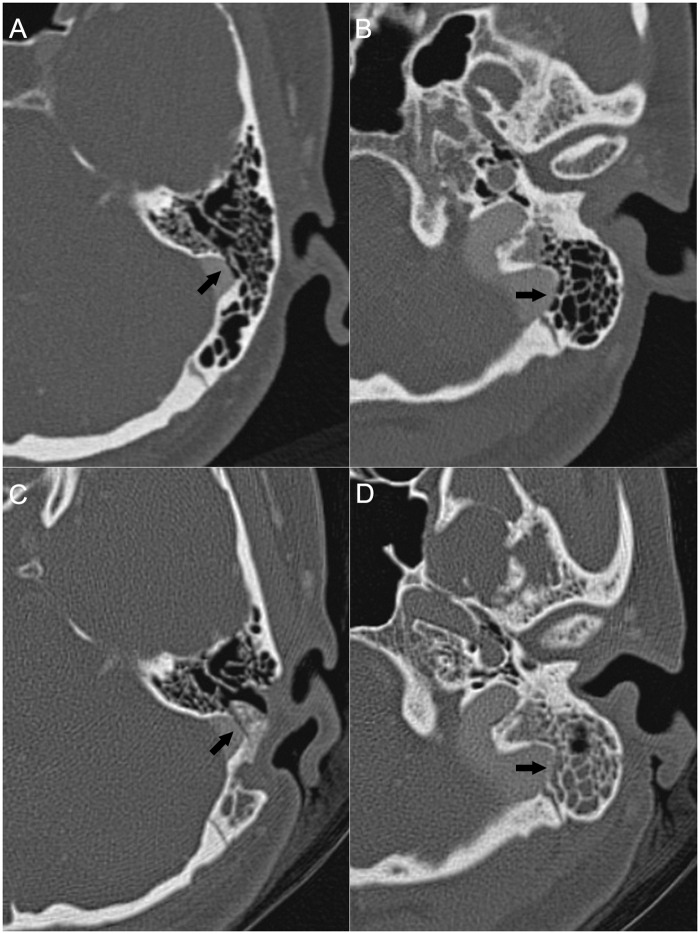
Preoperative (A, B) and postoperative (C, D) CTA images of a patient (Patient 16) with no change in PT following surgery. The layers in (A) and (B) correspond to those in (C) and (D), respectively. As shown in (C) and (D), the dehiscence in layer (A) is resurfaced, whereas that in the layer of (B) is not. A mastoid effusion is found postoperatively (D).
